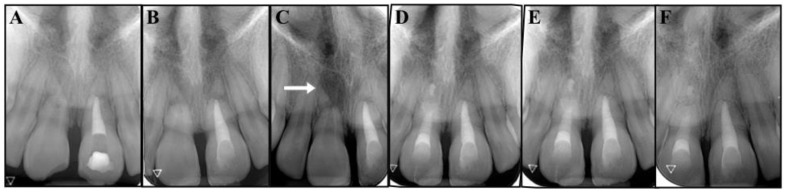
(A) Root canal filling with of tooth #2.1. (B) Post obturation and restoration of tooth #2.1. (C) The 6-month follow-up periapical radiograph reveals the radiolucency of tooth #1.1 (arrow). (D) Root canal filling with Portland cement and resin restoration of tooth #1.1. (E) Image from the 12-month follow-up. (F) Image from the 30-month follow-up.
